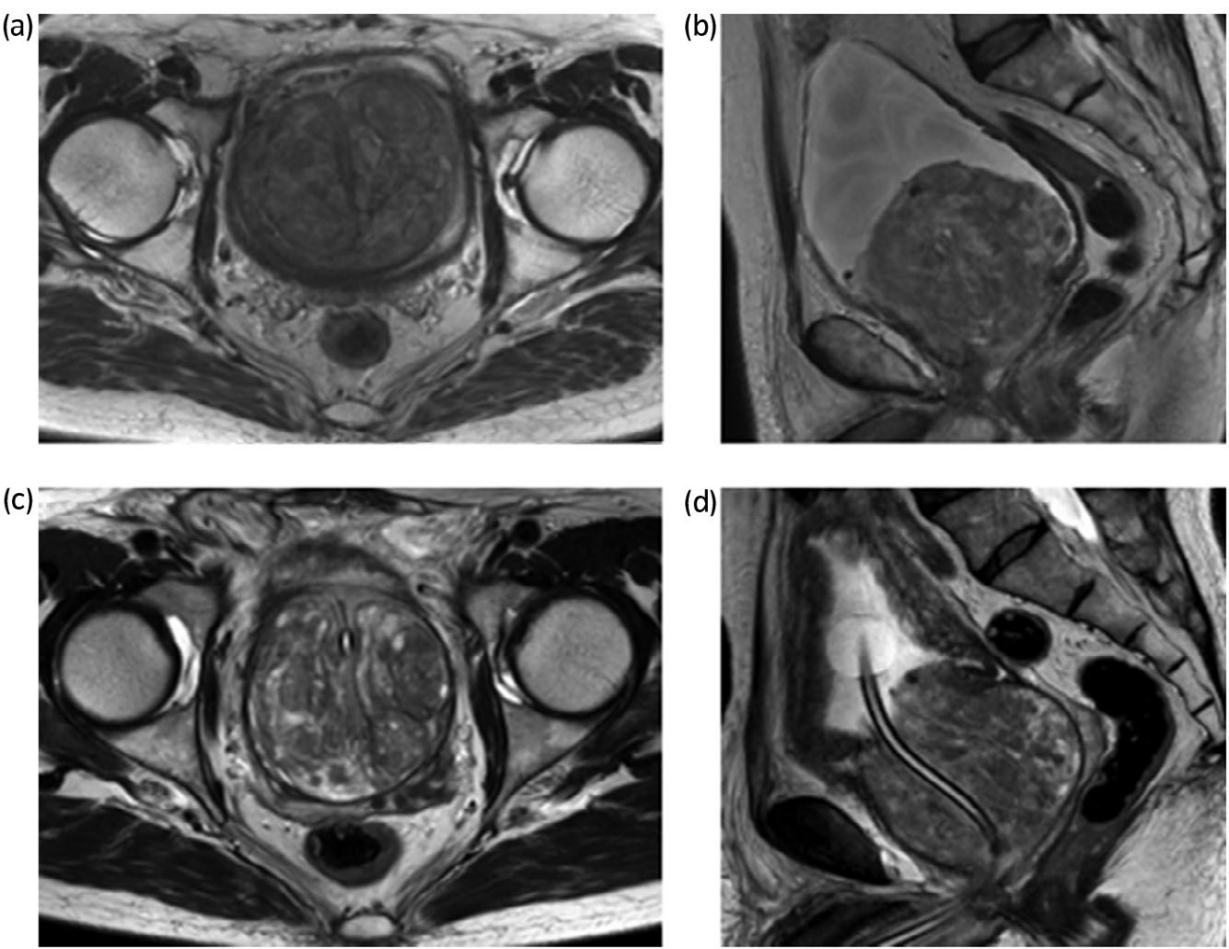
(a) An axial section of an MRI T2‐weighted image. (This is a 77‐year‐old case.) (b) A sagittal section of an MRI T2‐weighted image. (This is a 77‐year‐old case.) (c) An axial section of an MRI T2‐weighted image. (This is a 69‐year‐old case.) (d) A sagittal section of an MRI T2‐weighted image. (This is a 69‐year‐old case).
